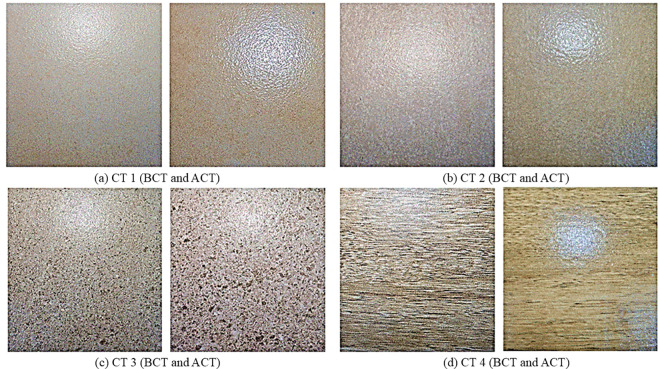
Photographic images of ceramic tiles for the dynamic friction tests before coating treatments (BCT) and after coating treatments (ACT): (a) CT 1 (indoor use), (b) CT 2 (indoor use), (c) CT 3 (outdoor use), and (d) CT 4 (outdoor use).
